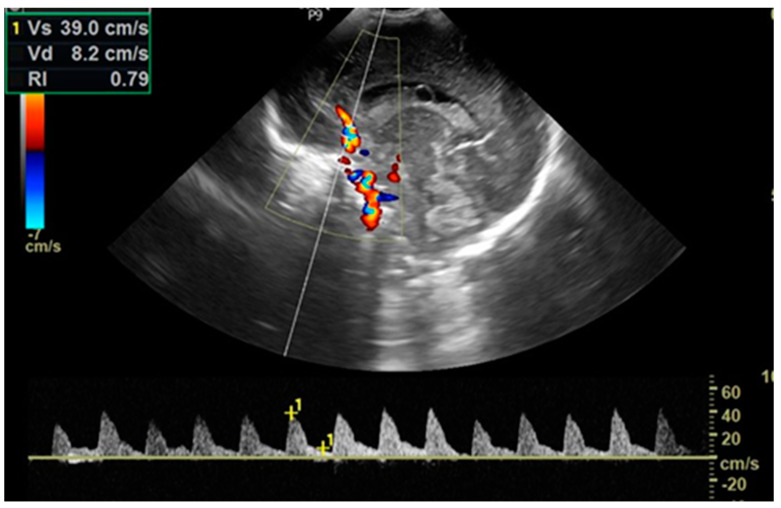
Doppler investigation of Anterior Cerebral Artery (ACA).
